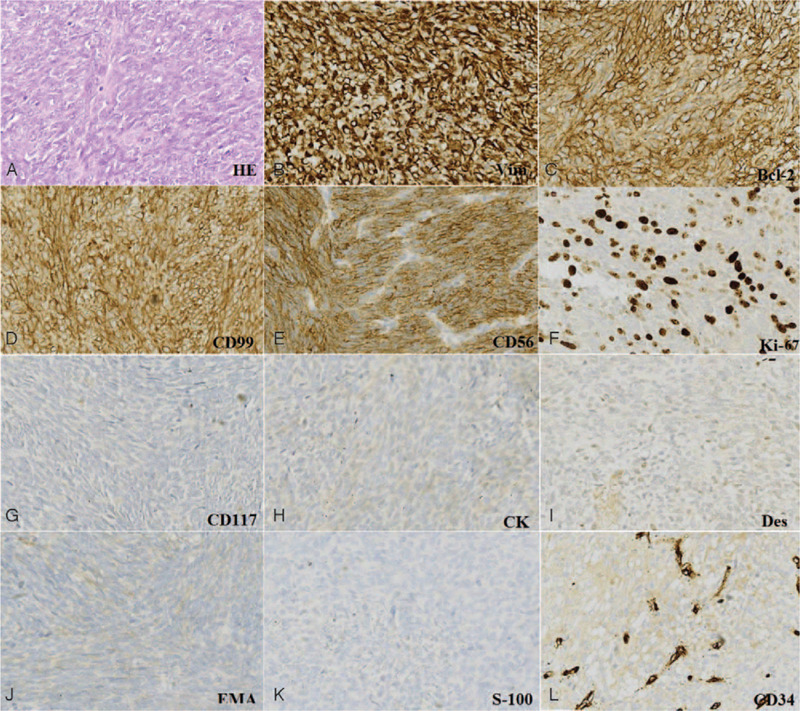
Preoperative magnetic resonance imaging of the lumbar spine before the first surgery (A and B) and postoperative x-ray imaging after the second surgery (C and D).
